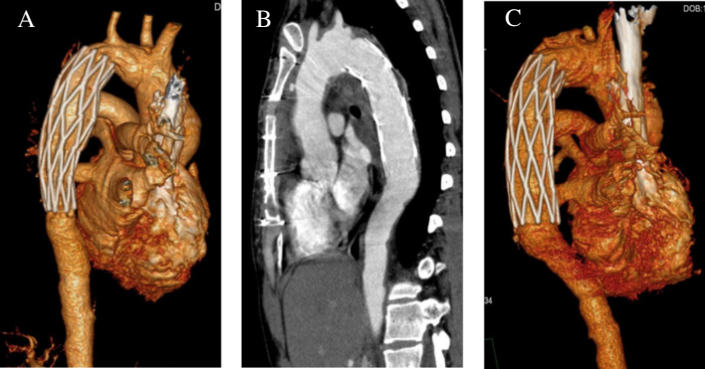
Computed tomography angiography images of postoperation. Its confirmed successful tamponade of the fistula before discharge (A) and after surgery for 6 months (B and C).
